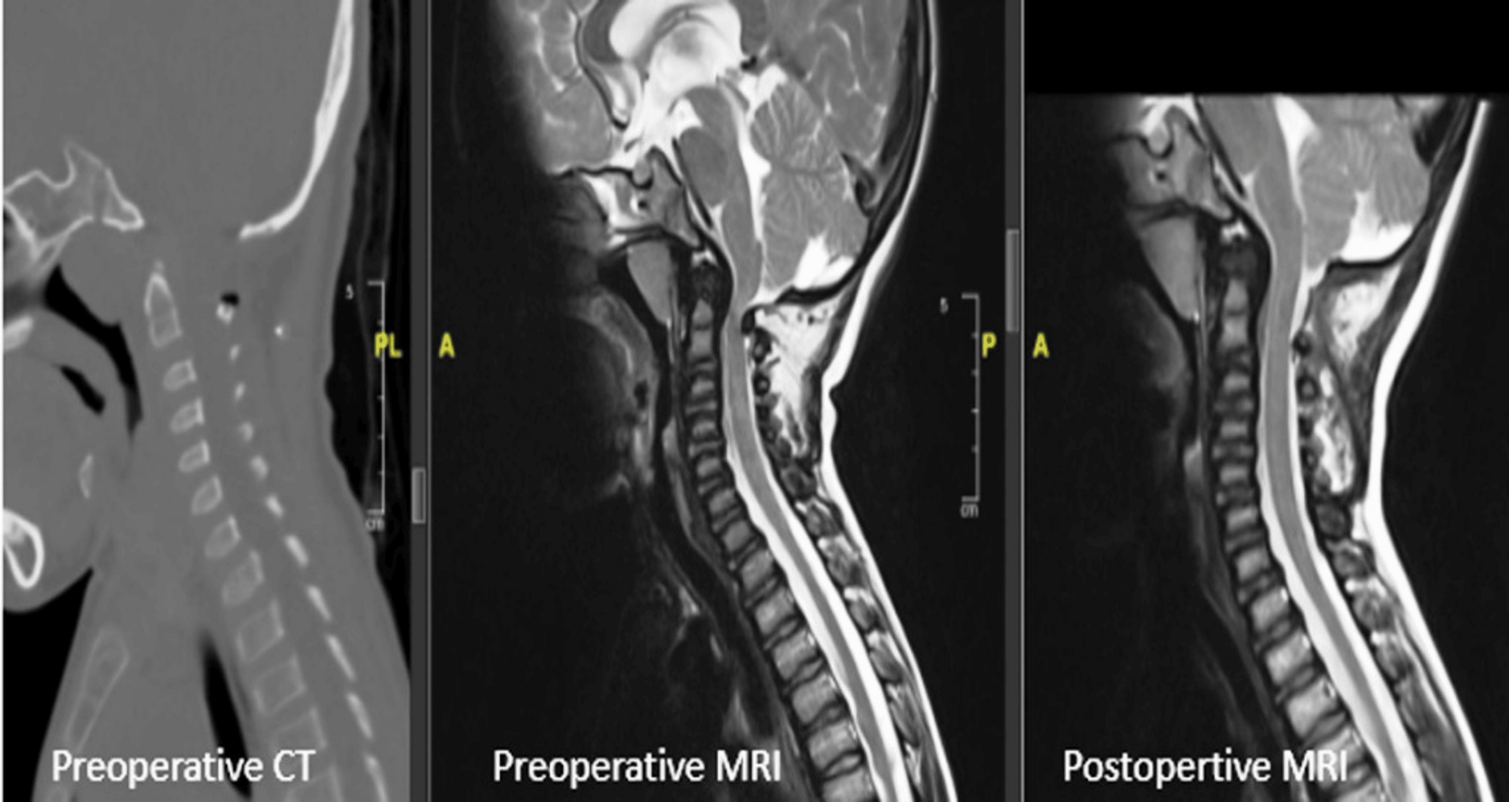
Case 2: Preoperative and postoperative CT and MRI images of the cervical spine showing adequate cervicomedullary decompression.
